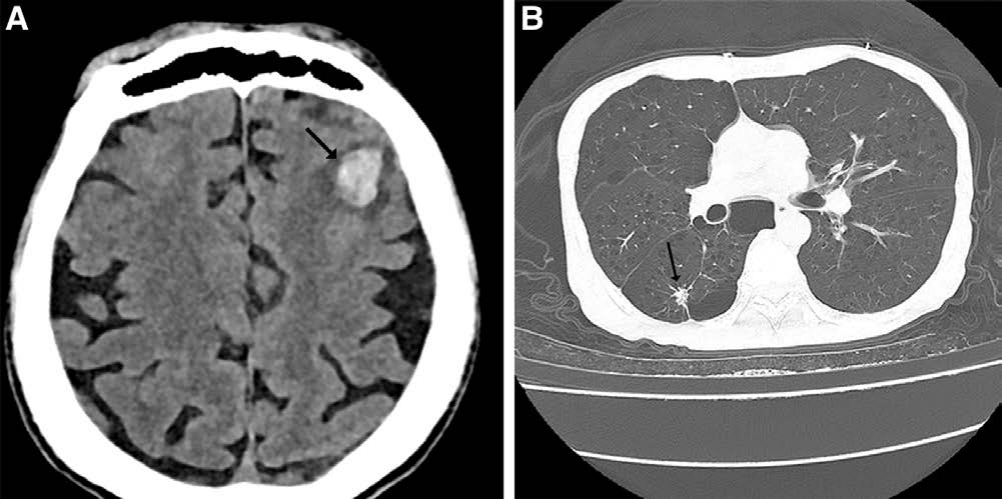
Computed tomography (CT) features in brain and chest. (A) Brain CT demonstrating small hematoma in left frontal lobe with peripheral cerebral edema. (B) Chest CT demonstrating chronic bronchitis, emphysema, and bullae in the right lung.
